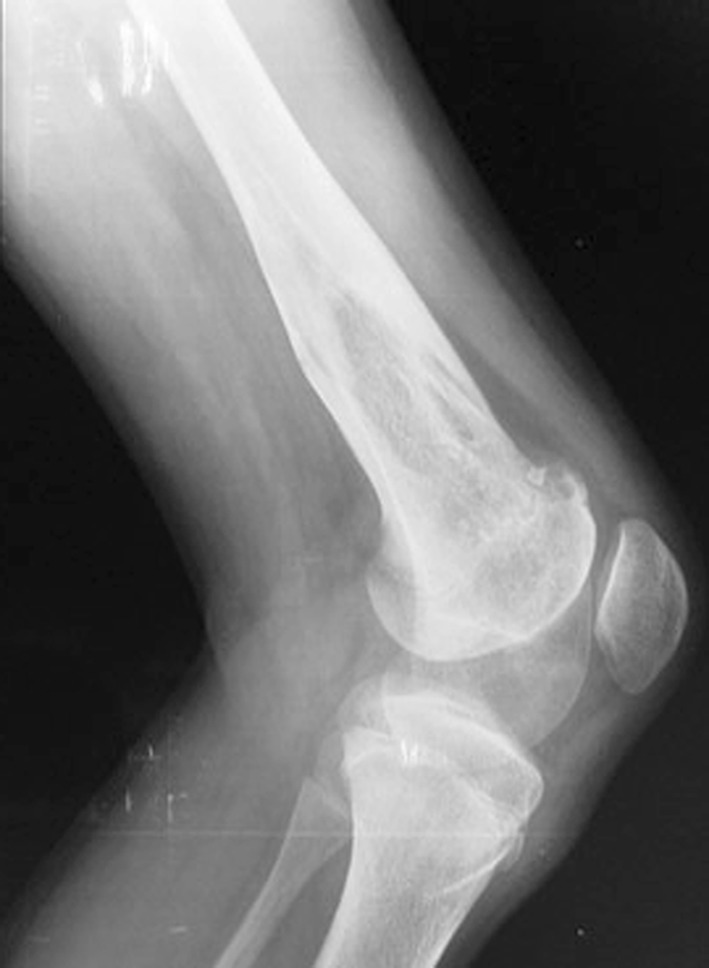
Lateral X-ray of the same patient
